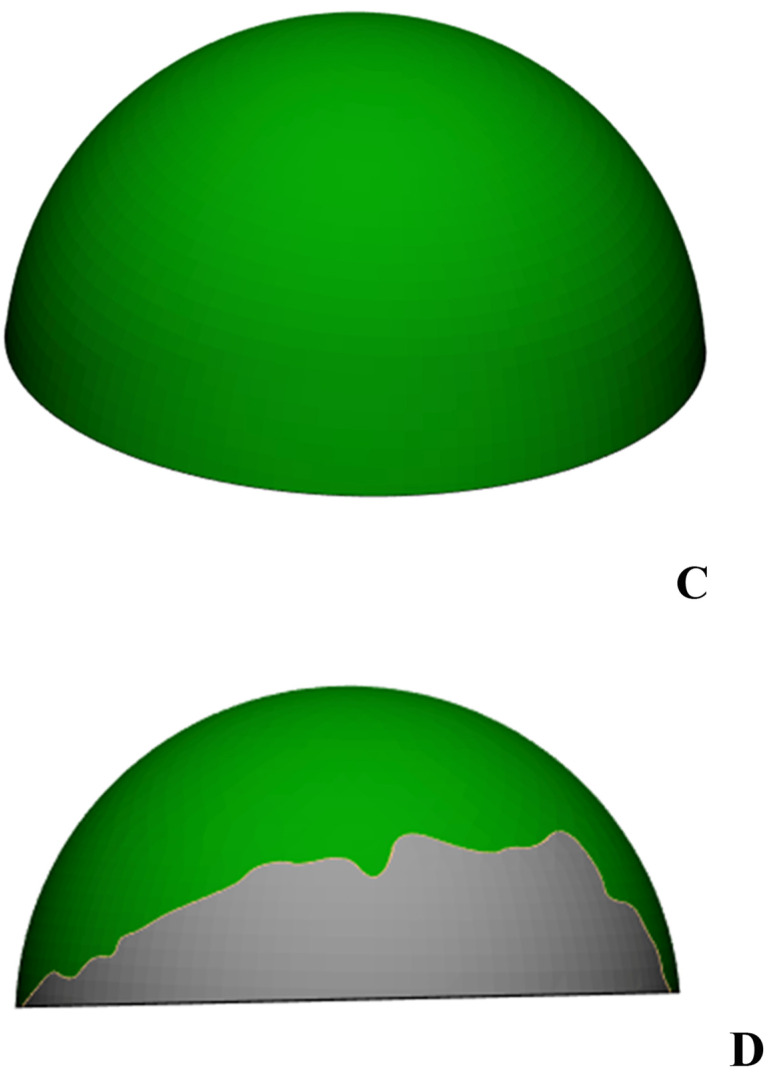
Measurement of 3D CT-based host bone coverage. (A) Recognize and cluster the serial outlines of pelvic bone and acetabular component structure. (B) Surface reconstruction images were achieved. (C,D) After reconstruction of the 3D image, the whole surface of acetabular cup and uncovered surface of cup was calculated.
